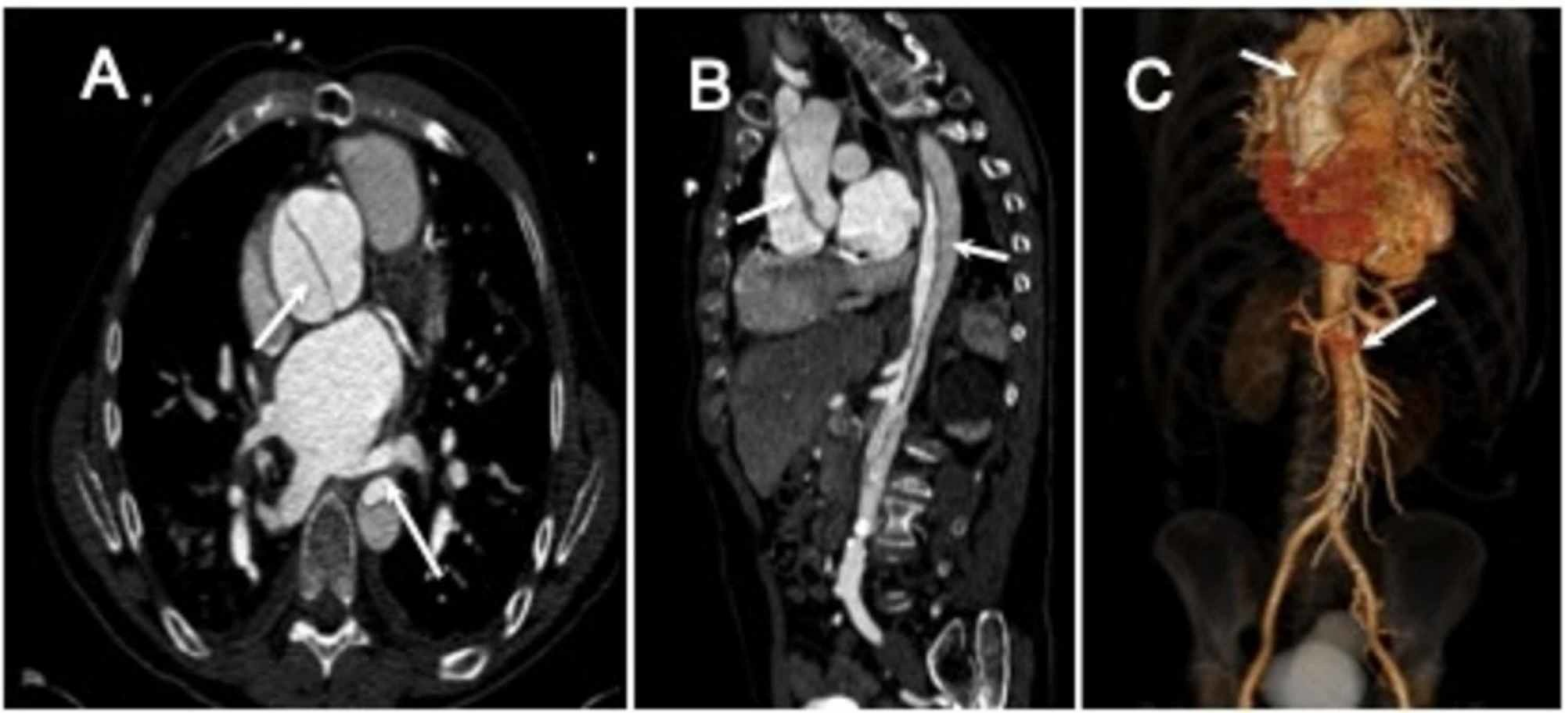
Type A Aortic Dissection A. Axial contrast-enhanced CT image demonstrating an intimal flap (arrows) consistent with a Stanford Type A dissection with extension into the distal thoracic aorta. B. 3D volume rendering image of the thoracic aorta visualization of the type A dissection (arrows). C. Sagittal reformat image shows the extension of the intimal flap into the abdominal aorta (arrows). The false lumen hypoattenuating as compared to the true lumen.
